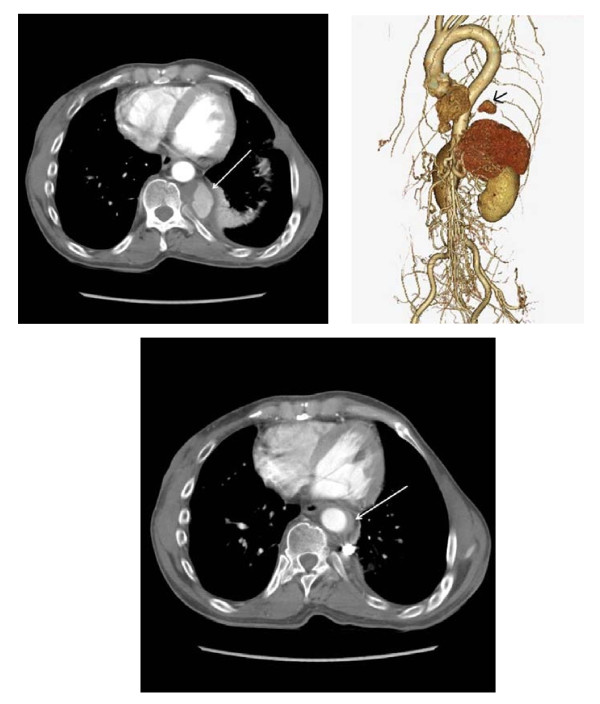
CT. CT showing massive intercostal aneurysm with thrombus (arrow) and mild pleural effusion (A,B). Post coil embolization CT showing early thrombosed Stanford type B acute aortic dissection (arrow).
